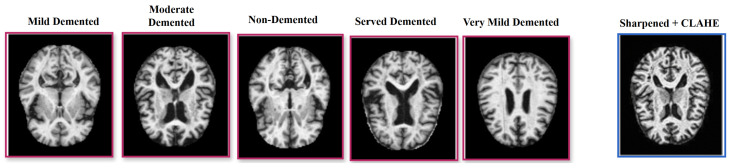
T1-weighted MRI samples of five Alzheimer’s disease (AD) classes with red borders (left) and a single preprocessed image with a blue border, enhanced using sharpening and CLAHE (right).
